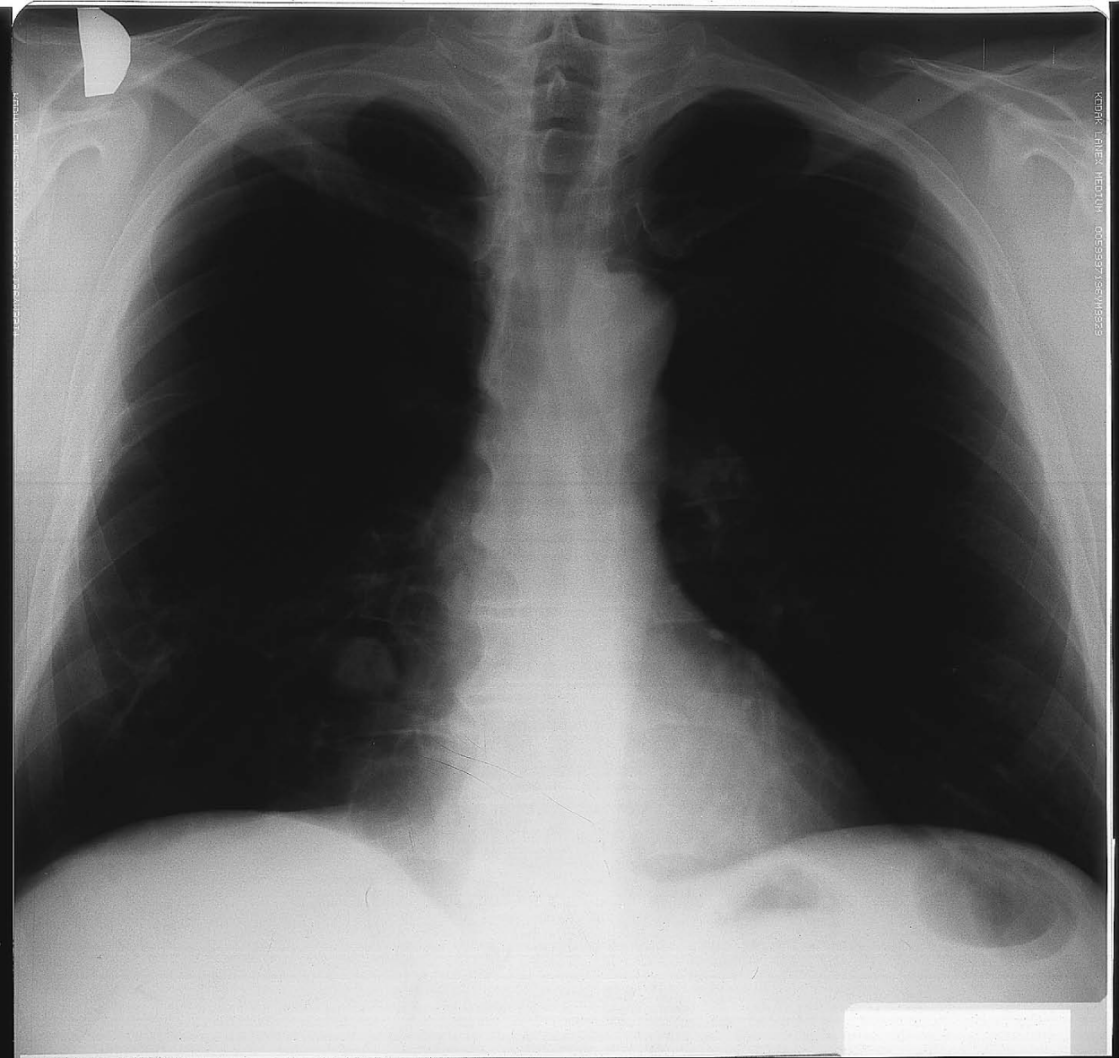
A cavitary lesion with air crescent in the right lower lobe seen on chest radiograph is characteristic of an aspergilloma.
